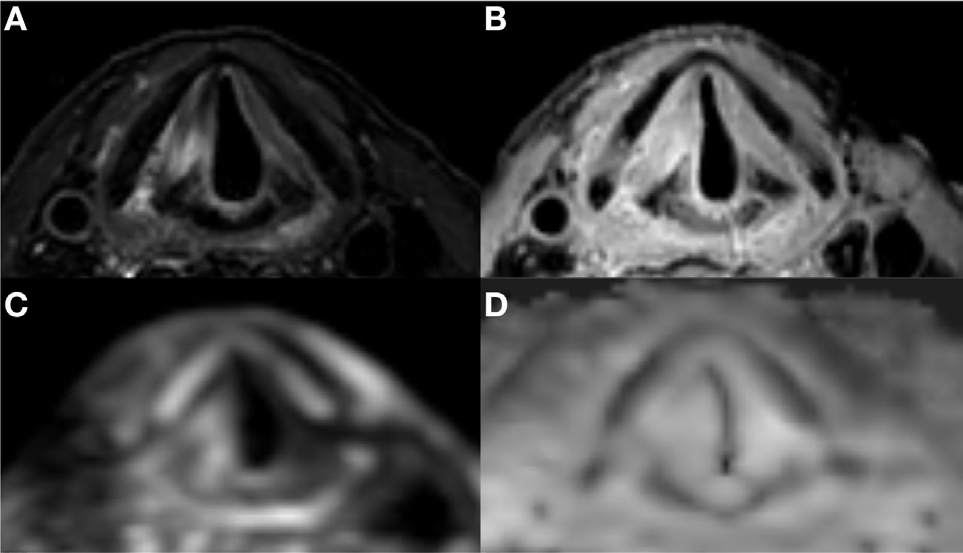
Laryngeal magnetic resonance imaging of a patient with superficial recurrence after radiotherapy of an early glottis carcinoma: high signal intensity in the right vocal cord and posterior paraglottic space on T2 (A) combined with a high signal intensity on both DWI (C) and ADC (D) is compatible with post irradiation edema and rules out deep tumor extension. There is diffuse enhancement on contrast enhanced T1 with fat suppression (B).
